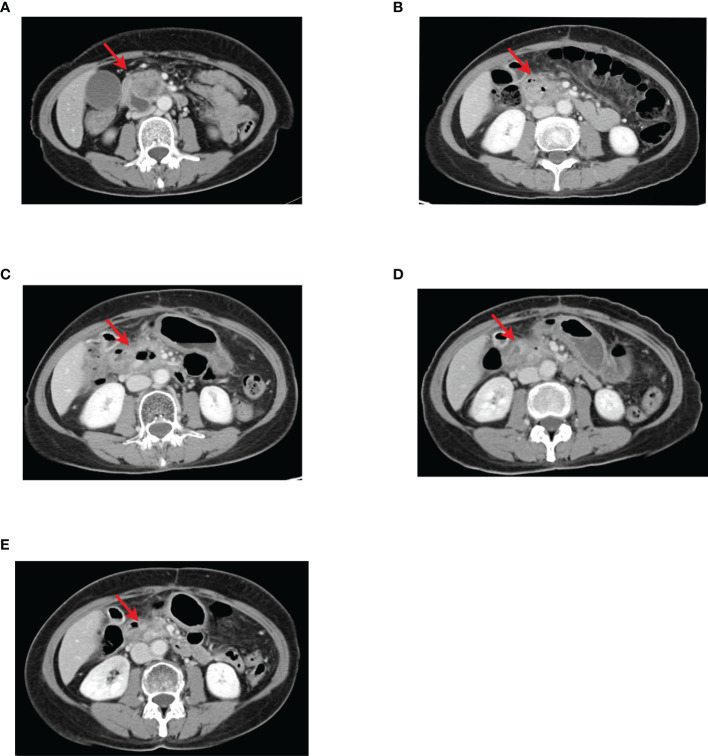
CT images of pancreatic lesions during concurrent chemo-radiotherapy followed by sequential chemotherapy, with red arrows indicating the location of pancreatic head lesions. (A) The initial CT examination revealed a mass in the head of the pancreas, about 3.6 × 3.5 cm. (B) After concurrent chemo-radiotherapy, the pancreatic head cancer lesion did not change much compared with the previous, about 3.9 × 3.3 cm. (C–E) One to six cycles of sequential chemotherapy with gemcitabine + TS-1 gradually reduced the pancreatic head lesion and the efficacy evaluation was PR.
